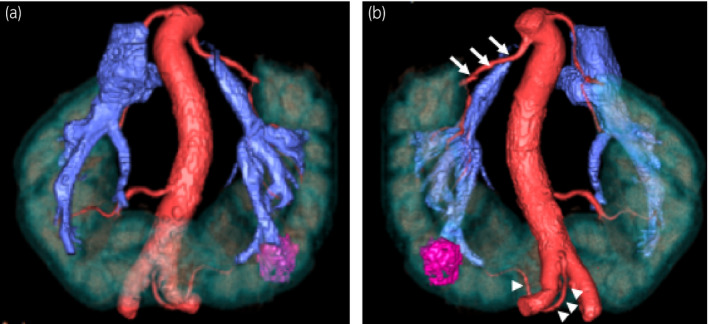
Three‐dimensional CT provided more detailed vessel information. There were two renal arteries and the tumor was located in a posterolateral region. One artery arose from the normal anatomic location (arrow) and the other arose from the dorsal side of the aortic bifurcation and snaked into the ventral side to nourish the tumor and isthmus (arrow head). (a) Anterior view. (b) Posterior view revealed the renal artery arising from the aortic bifurcation was also considered to be easily accessible via a retroperitoneal approach
